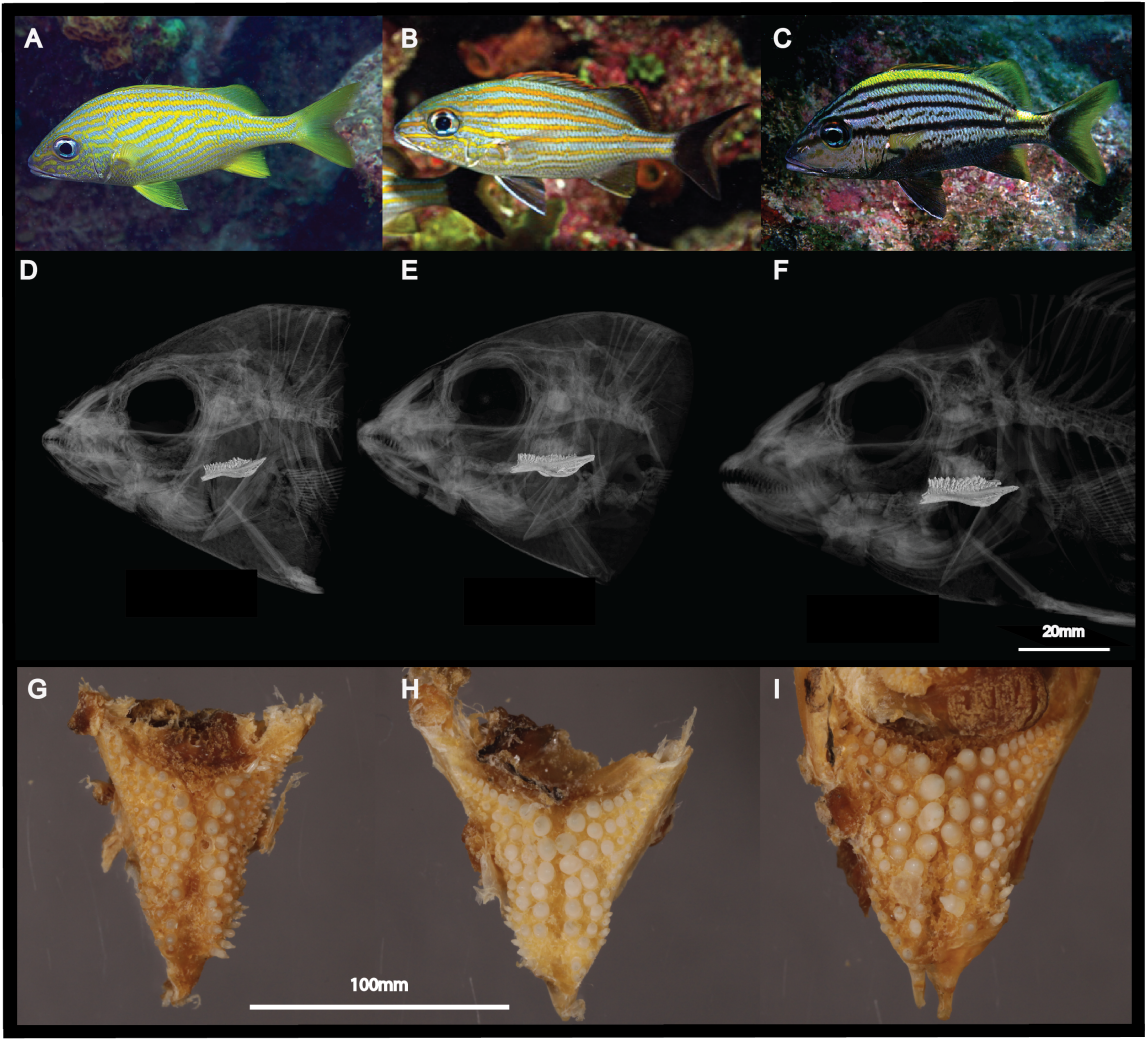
Morphological differences between the three sympatric species of Haemulon. Grunts are characterized by differences in their head and pharyngeal morphology, which are potentially associated with their feeding preferences. This figure shows photographs of H. flavolineatum (A), H. carbonarium (B) and H. macrostomum (C) in the wild; computed tomography scans of the cranium (D–F); and photographs of the lower pharyngeal teeth (G–I). Image credit: LA Rocha, EL Stanley, MA Bernal.
